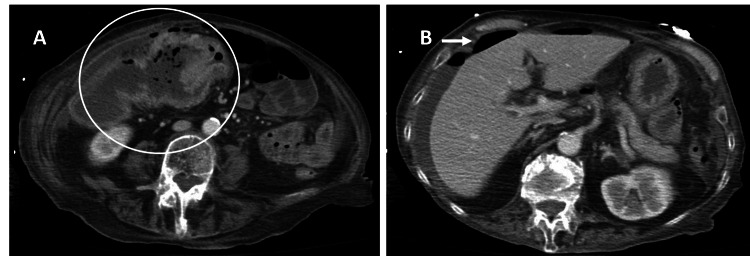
CT scan of the abdomen and pelvis showing (A) location of mass in the transverse colon (circle) and (B) the free air under diaphragm (arrow) concerning for perforation.
